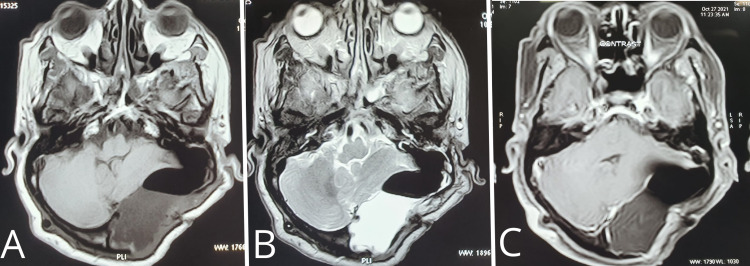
Postoperative MRI of T1-weighted axial section (A), T2-weighted axial section (B), T1-weighted contrast-axial section (C).
